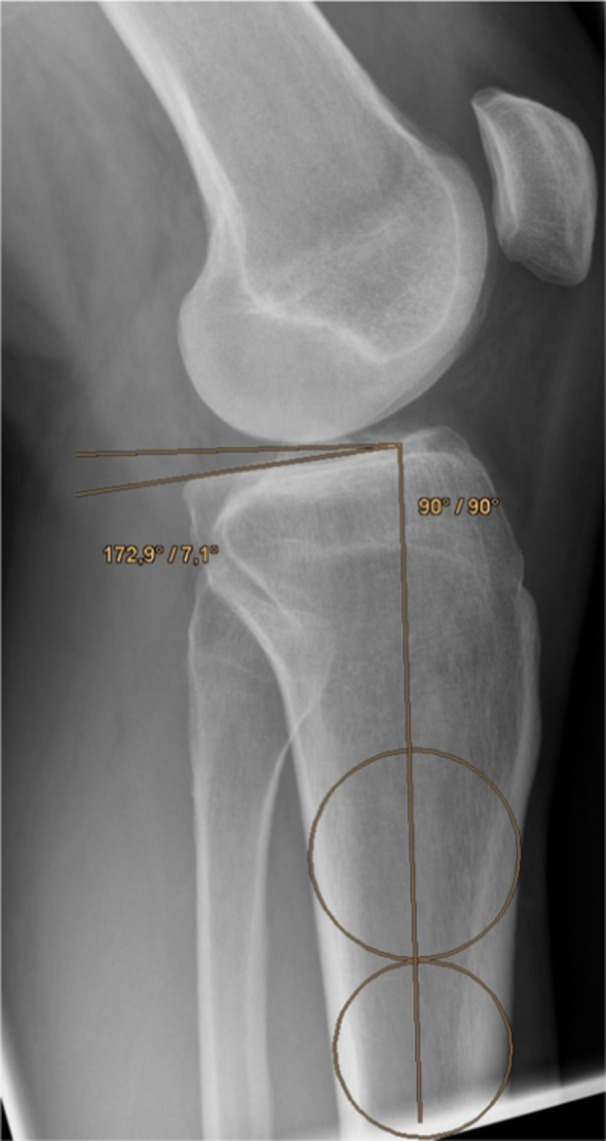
Radiographic assessment on lateral knee radiograph.
